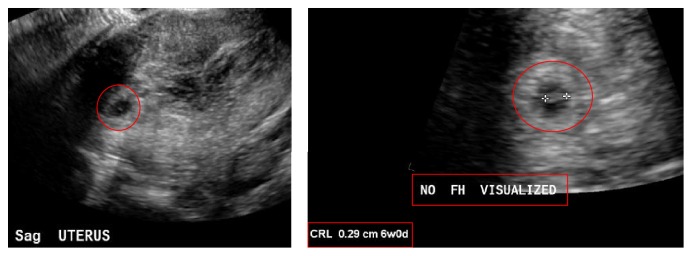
Patient number 2. Obstetric ultrasound on 3/2/2014: intrauterine gestational sac seen. There is adjacent heterogeneity suggesting subchorionic hemorrhage. No fetal cardiac activity detected. Fetal pole measures approximately 2.9 mm.
